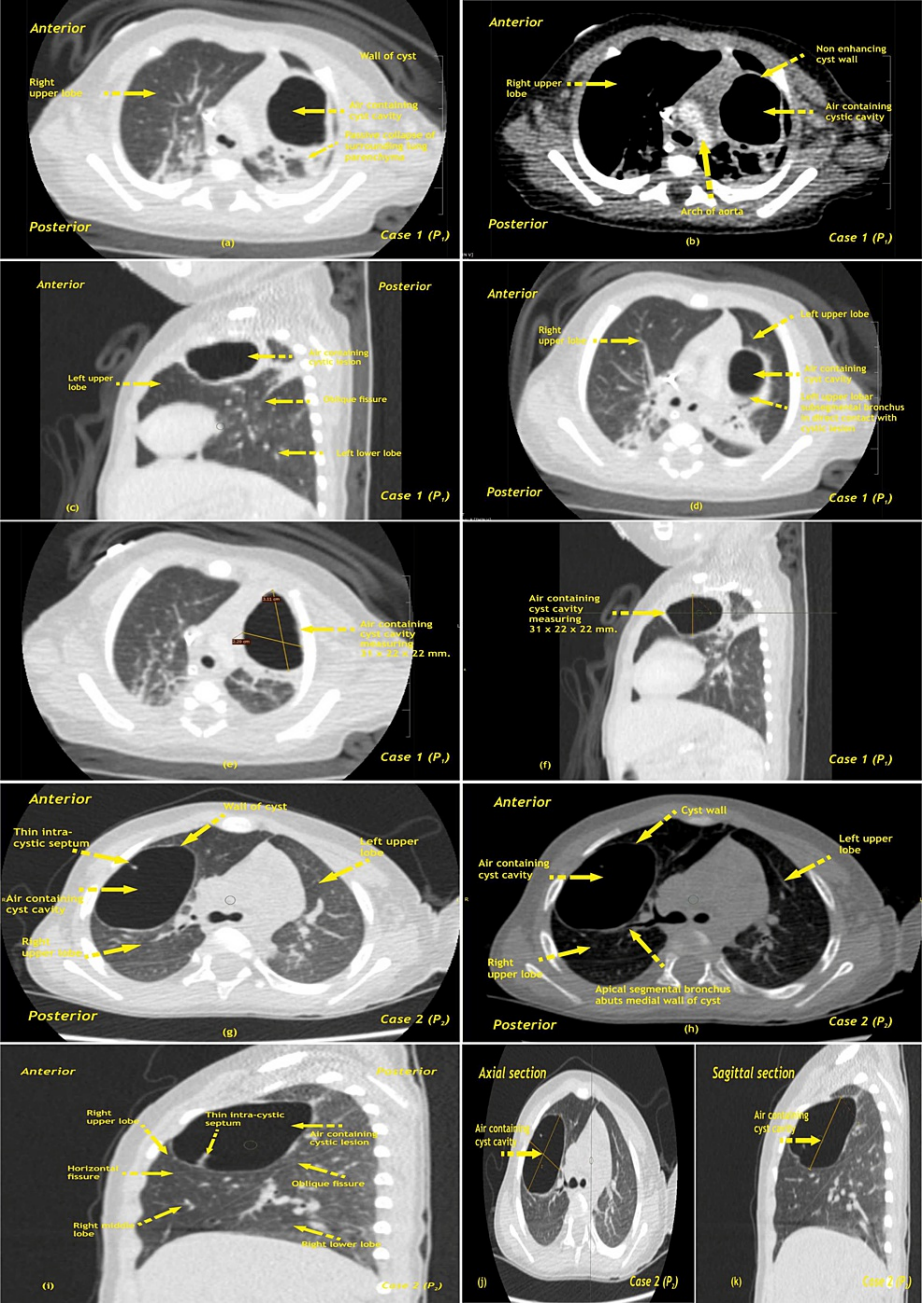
Case 1 (P1) (a) CECT scan of chest axial section in lung window (section 1) at the level of upper lobe showing a well-defined, thin-walled air containing cystic cavity lesion with surrounding lung parenchyma shows changes of passive collapse in left lung upper lobe. (b) CECT of section 1 in mediastinal window at level of upper lobe showing well-defined air-containing cavernous lesion with thin wall on CECT scan. (c) CECT of chest sagittal section in lung window showing air containing well-defined cystic lesion noted in left upper lobe with adjacent areas of collapse and left oblique fissure which separate left upper and lower lobe. (d) CECT of (section 1) in lung window at level of upper lobe showing a well-defined air-containing cavernous lesion in left upper lobe with upper lobar subsegmental bronchus appear in direct contact with cystic lesion and adjacent areas of passive collapse. (e) CECT of (section 1) showing well-defined air-containing cystic lesion in upper lobe of left lung measuring approximately 31 x 22 x 21 mm suggests CPAM (Type I) more likely. (f) CECT of chest sagittal section showing well-defined air-containing cystic lesion in upper lobe of left lung measuring approximately 31 x 22 x 21 mm suggests CPAM (Type I) more likely. Case 2 (P2) (g) HRCT scan of (section 1) at level of upper lobe showing a big, well-defined, oval, thin-walled, air-containing cystic cavity lesion in anterior segment of right upper lobe. There is thin oblique internal septation noted in antero-inferior portion of cavity. (h) HRCT scan of (section 1) in mediastinal window at level of upper lobe showing a big, well-defined, oval, thin walled, air containing cystic cavity lesion in anterior segment of right upper lobe. Apical segmental bronchus abuts medial wall of cystic lesion. (i) HRCT scan of chest sagittal section in lung window at right lung showing a big, well-defined, oval, thin-walled, air-containing cystic cavity lesion in anterior segment of right upper lobe. There is thin oblique internal septation noted in antero-inferior portion of cavity. The cystic lesion also abuts posteriorly to oblique fissure and inferiorly to horizontal fissure. (j & k) HRCT scan of chest axial and sagittal sections showing big, well-defined, oval, thin-walled, air-containing cystic lesion in anterior segment of right upper lobe measuring approximately 52.5 x 36.6 x 41.7 mm (AP X TX X SI) suggests congenital pulmonary airway malformation (Type I) more likely. CECT - Contrast-enhanced computed tomography; HRCT - High-resolution computed tomography; CPAM - Congenital pulmonary airway malformation; AP - Antero-posterior; TX - Transverse; SI - Supero-inferior; P - Patient Image Credit: Dr. Dharmendra B. Parmar
